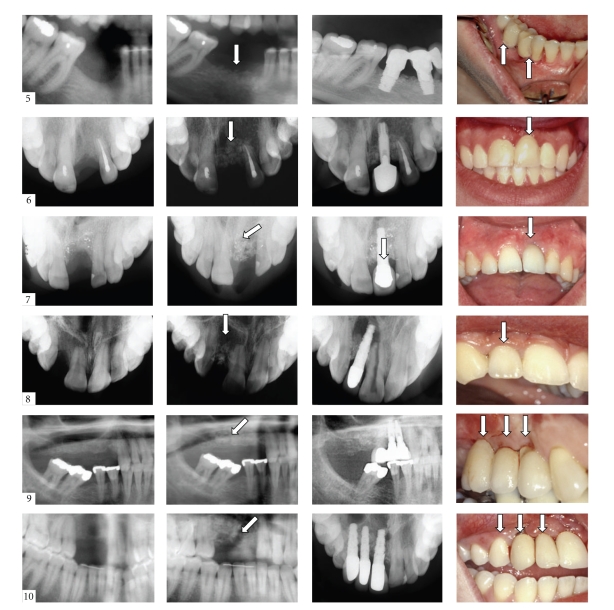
Overview for patients 5–10 from the study by Meijer et al. to reconstruct a maxillary defect and placement of dental implants. First column; radiographs showing the alveolar defects. Second column; showing the reconstruction (arrow) by augmentation (5–8) and by sinus elevation procedure (9 and 10). Third column; radiographs showing the dental implants and the prosthetic construction (crown or bridge). Fourth column; clinical situation at the end of the rehabilitations (arrow).
